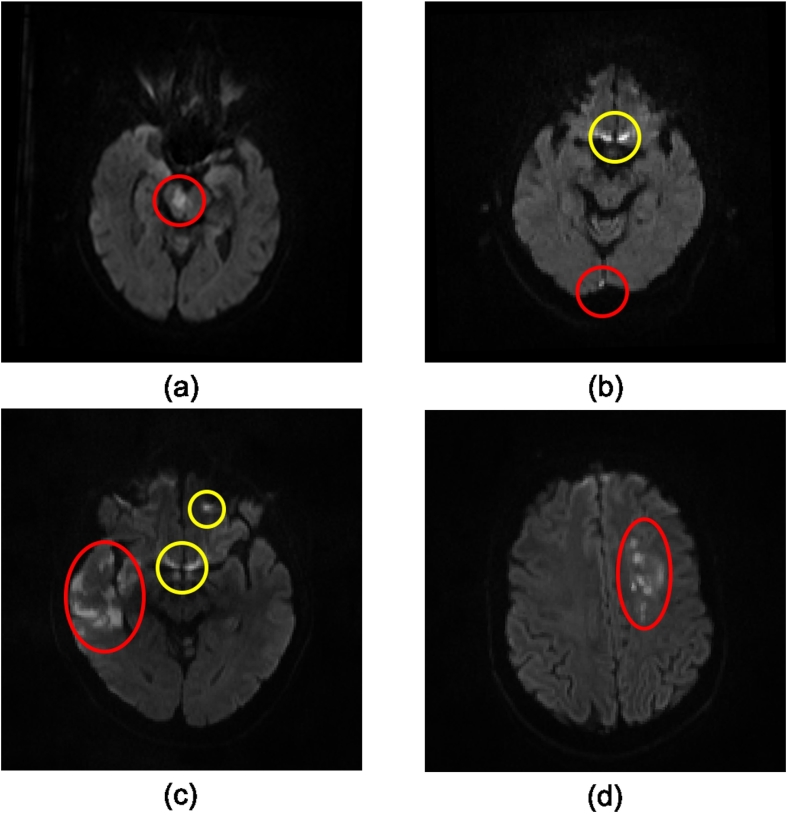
Examples of acute ischemic lesions in DWI. The red circles indicate the acute ischemic lesions and the yellow ones show the artefacts. (For interpretation of the references to color in this figure legend, the reader is referred to the web version of this article.)
